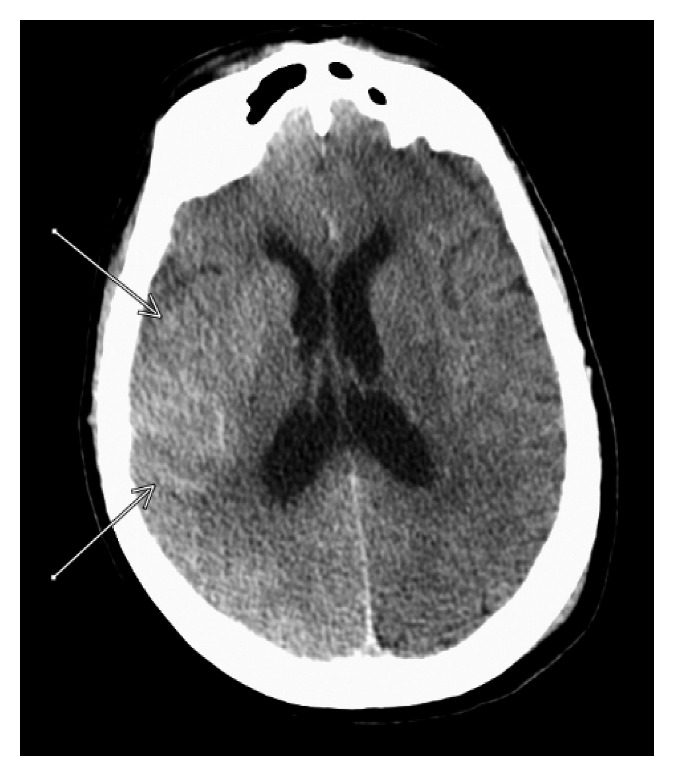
CT of the brain on POD one showed decreased hyperintensity on the right side of the cortex compared to the CT of the brain immediately post-op (Figure 1).
